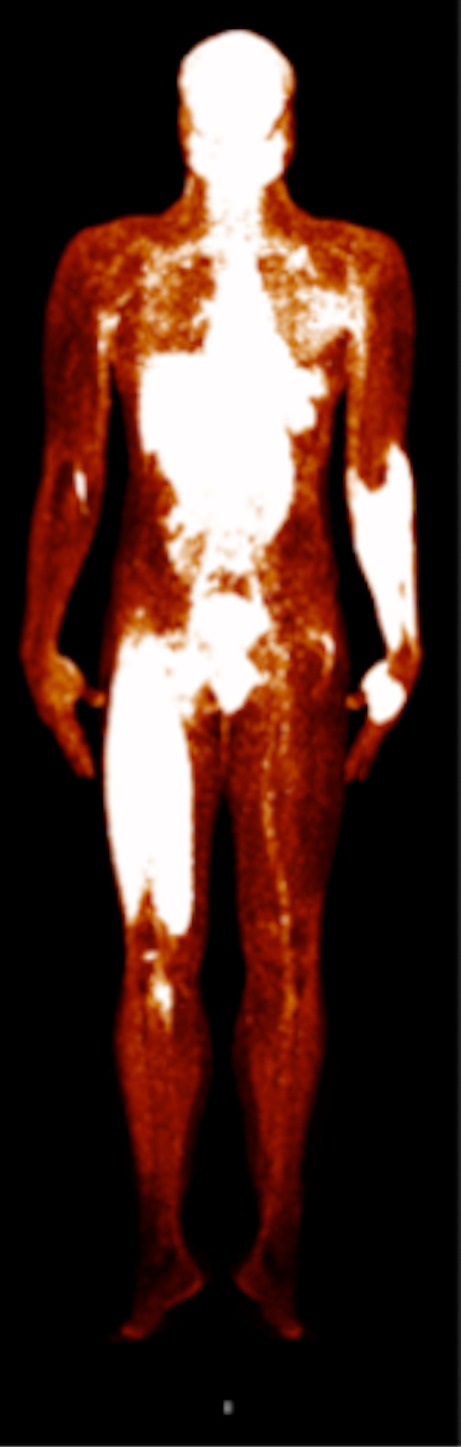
Whole-body ^18^F-FDG PET image of subject after the exercise intervention. The right quadriceps and lower left arm muscles used in the exercise intervention show significantly higher glucose uptake than the resting contralateral limbs
